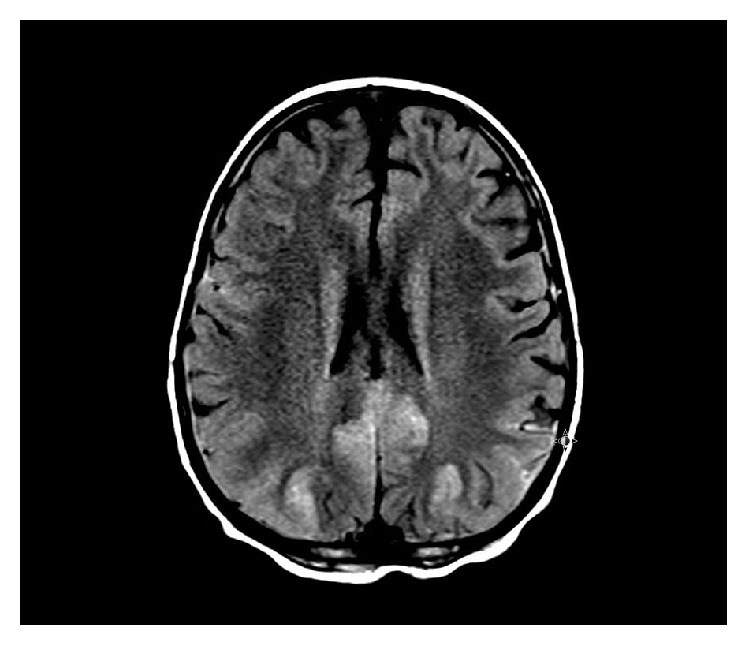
Brain MRI: T2 hyperintensity involving the cortex and possibly subcortical white matter at the occipital and posterior parietal lobes, bilaterally and fairly symmetrically.
